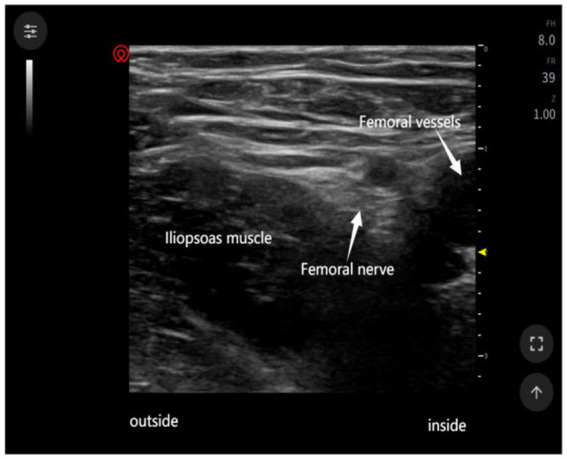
Ultrasound imaging of the FN.
